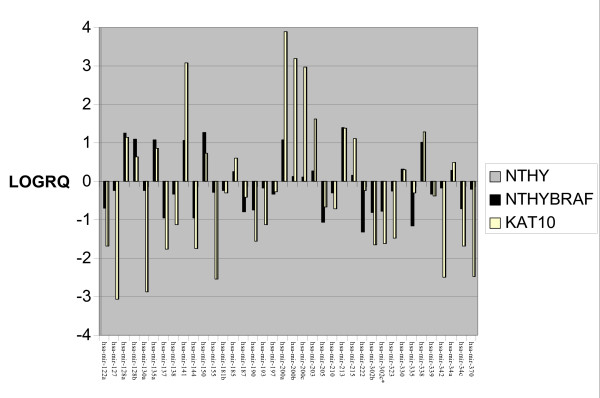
Differentially expressed miRNAs Between BRAF V600E harbouring Cell Lines and Normal Thyroid Cell line. Delta delta CT was performed using Nthy-ori 3-1 as a normal control. The log of the RQ values was used to plot the relative fold change of Nthy-BRAF and KAT10 against Nthy-ori 3-1. miRNAs are on the × axis
